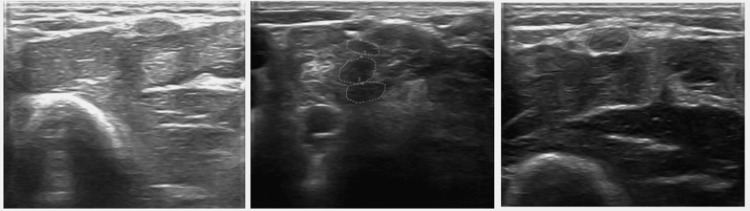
Ultrasound images Ultrasound images of the ulnar nerves using a 15 MHz transducer showing a significant increase in the CSA of the left and right ulnar nerves above the elbow. (Left image) Left ulnar nerve 10 cm above the elbow with a CSA of 16.9 mm ² (center image) thickening of the C5-C6-C7 roots with CSAs of 15.2 mm², 21.5 mm², and 18.7 mm², respectively. (Right image) Right ulnar nerve 10 cm above the elbow with a CSA of 21.6 mm².
